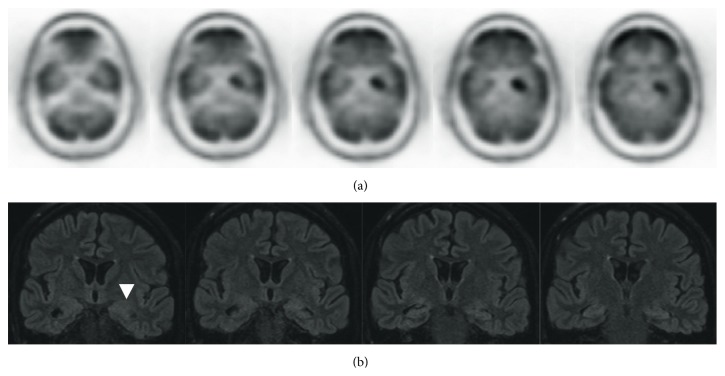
(a) Axial PET demonstrating significant uptake of FDG in the left mesial temporal lobe. (b) Coronal FLAIR MRI with subtle hyperintensity surrounding the left hippocampus (arrowhead).
